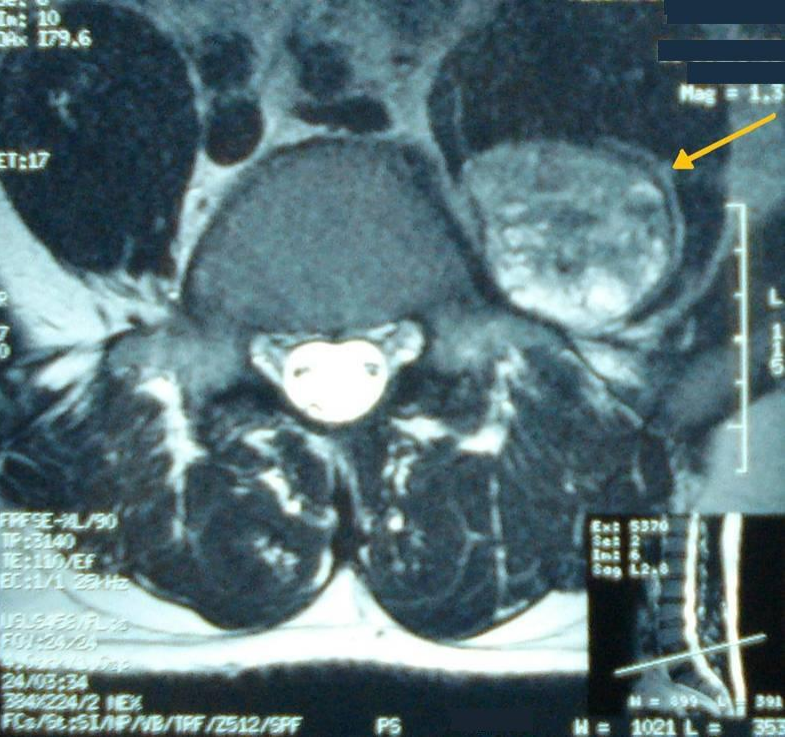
Lumbar spine MRI showing an encapsulated ovoidal retroperitoneal lesion in the L4-L5 level with scintigraphic uptake and cystic degeneration.
